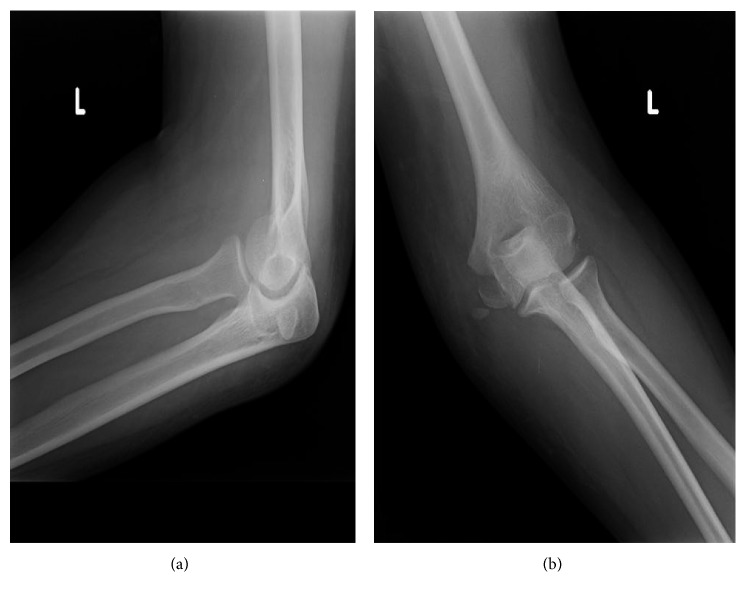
Anteroposterior and lateral radiographs of the left elbow joint after closed reduction showing a displaced medial epicondyle avulsion fracture.
